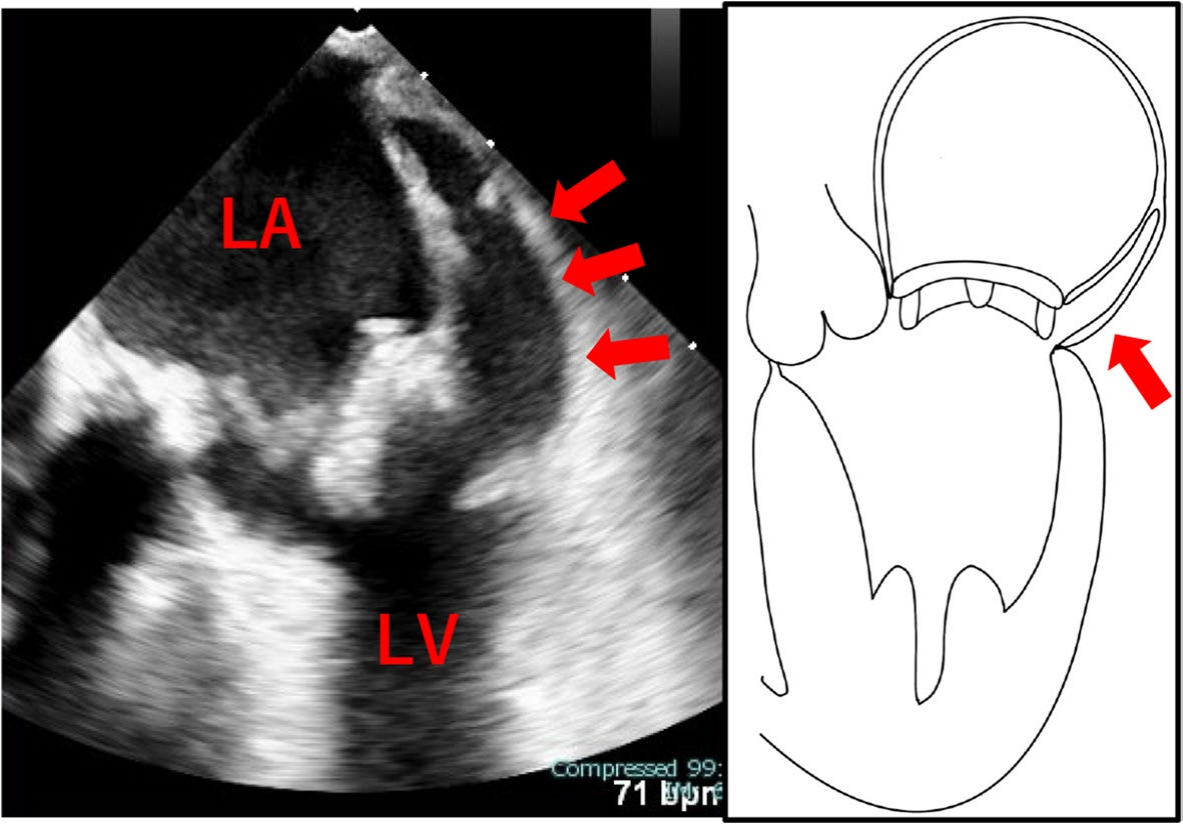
Transesophageal echocardiography (TEE) reveals the prosthetic valve partial detachment and a false lumen posterior side of left atrium, where blood flows into
